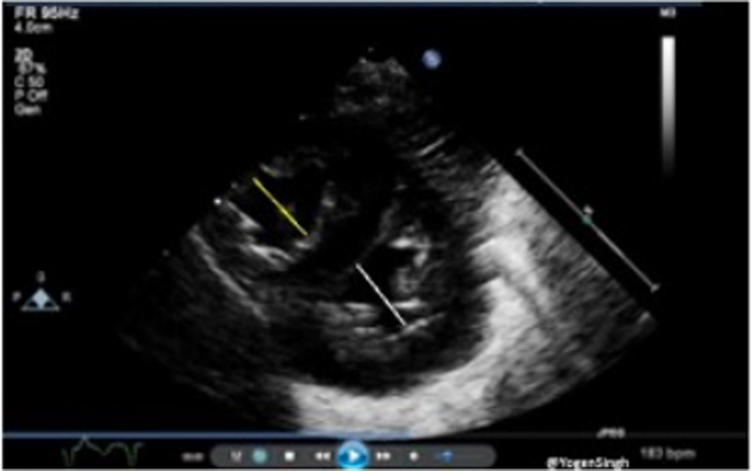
Right ventricle (RV) to left ventricle (LV) end-systolic ratio measurement in the parasternal short-axis view.
